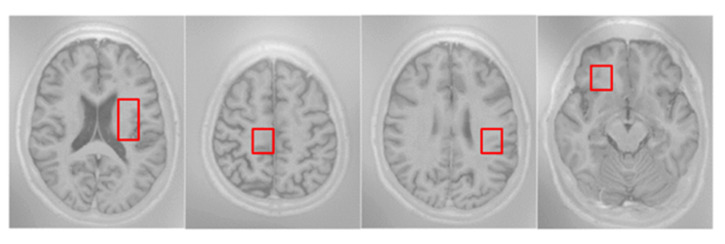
Example of the modality of T1_IR from patient no. 5 MRI. In this example, the intensities of different brain tissue in the different local brain regions are close to each other, like the examples in the red boxes.
